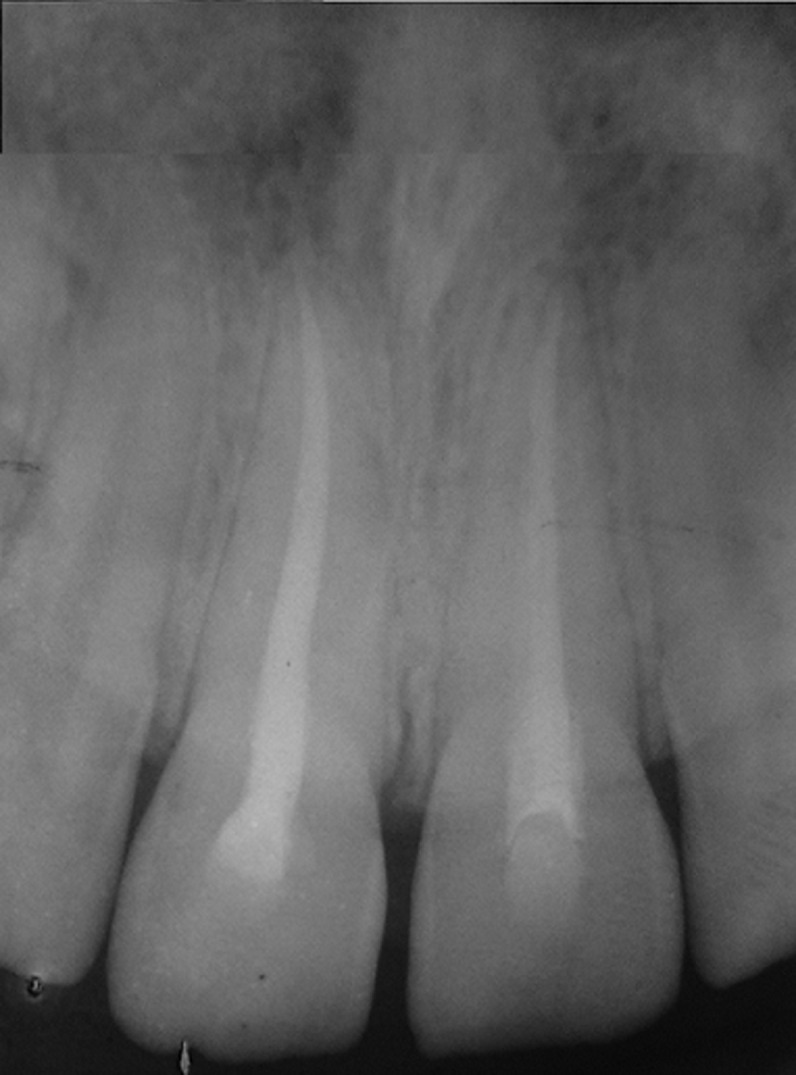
radiograph of maxillary incisors 2 years after injury
